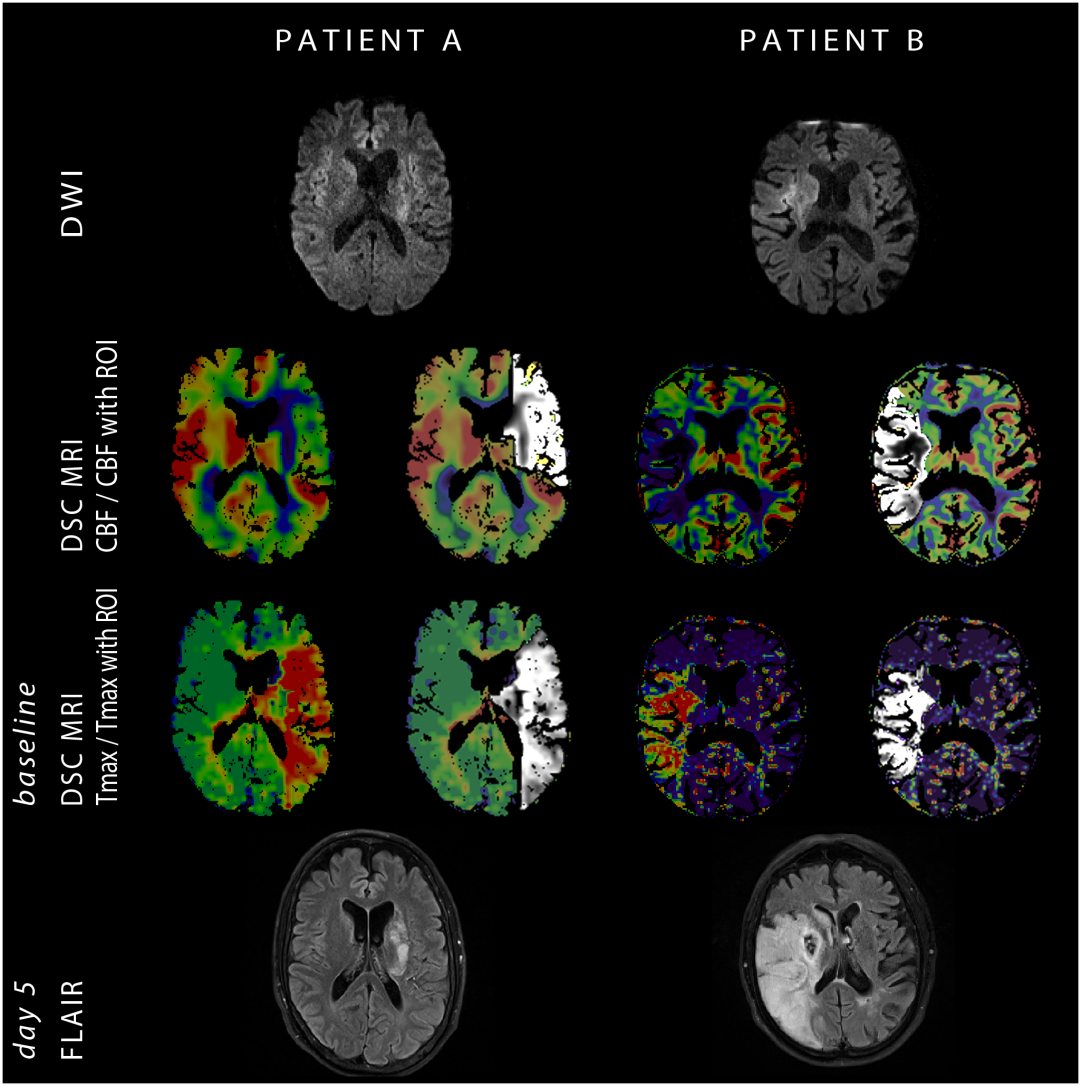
Comparison of two patients both imaged 1 hour after symptom onset with a proximal occlusion of the middle cerebral artery and a Higashida score of 2 on source DSC MRI. Patient A had a left-sided stroke with NIHSS at admission of 12, an initial infarct volume of 3.8ml, perfusion deficit of 200ml on Tmax4s and 108ml on CBF with a CBF/Tmax4s volume ratio of 0.54. The final infarct size was 15.5ml. Patient B had a right-sided stroke with NIHSS at admission of 19, an initial infarct volume of 5.8ml, perfusion deficit of 87ml on Tmax4s and 84ml on CBF with a CBF/Tmax4s volume ratio of 0.96. The final infarct size was 208ml.
